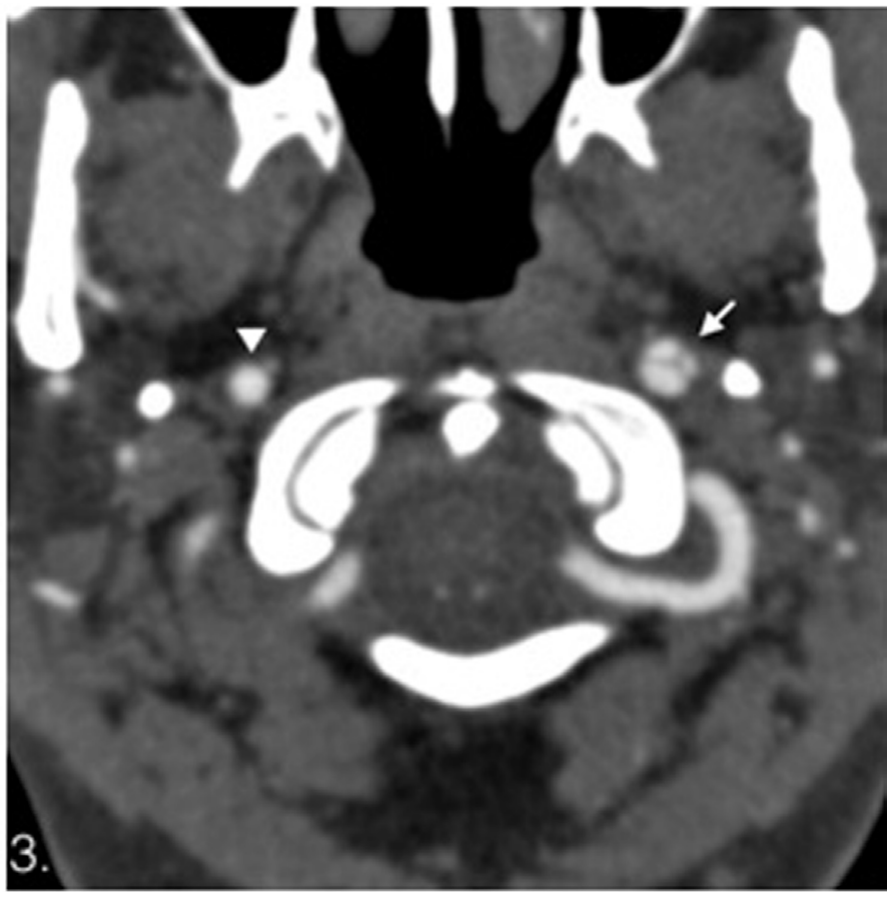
Computed tomography angiography. Dissection of the left internal carotid artery with flaps (white arrow) compare with normal right internal carotid artery (arrowhead). No abnormalities on the left vertebral artery are seen on this study.
